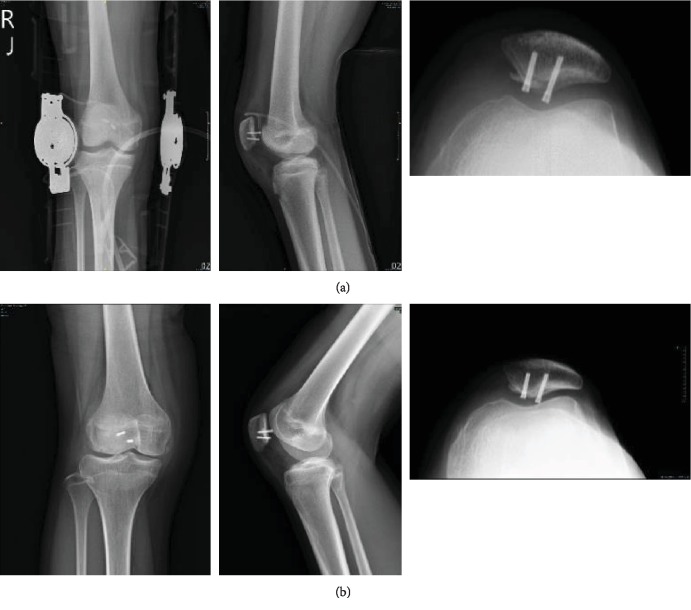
(a) Postoperative standard radiographs at the 1-week follow-up. Note that the dislocation and articular surface are corrected. (b) Postoperative standard radiograph showing a healed fracture site and the implant in situ at 9 months.
